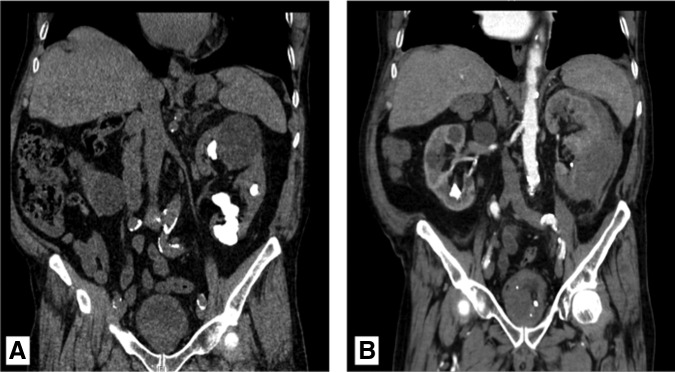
Case 1: Interval development of a left renal mass. (A) CT abdomen pelvis from July 2013 demonstrating stable stone burden compared to the original CT from August 2011 (not shown). (B) CT scan in September 2013 following PCNL, revealing a subcapsular hematoma and heterogeneous density in the left renal pelvis concerning for tumor *vs* blood clot. PCNL, percutaneous nephrolithotomy.
